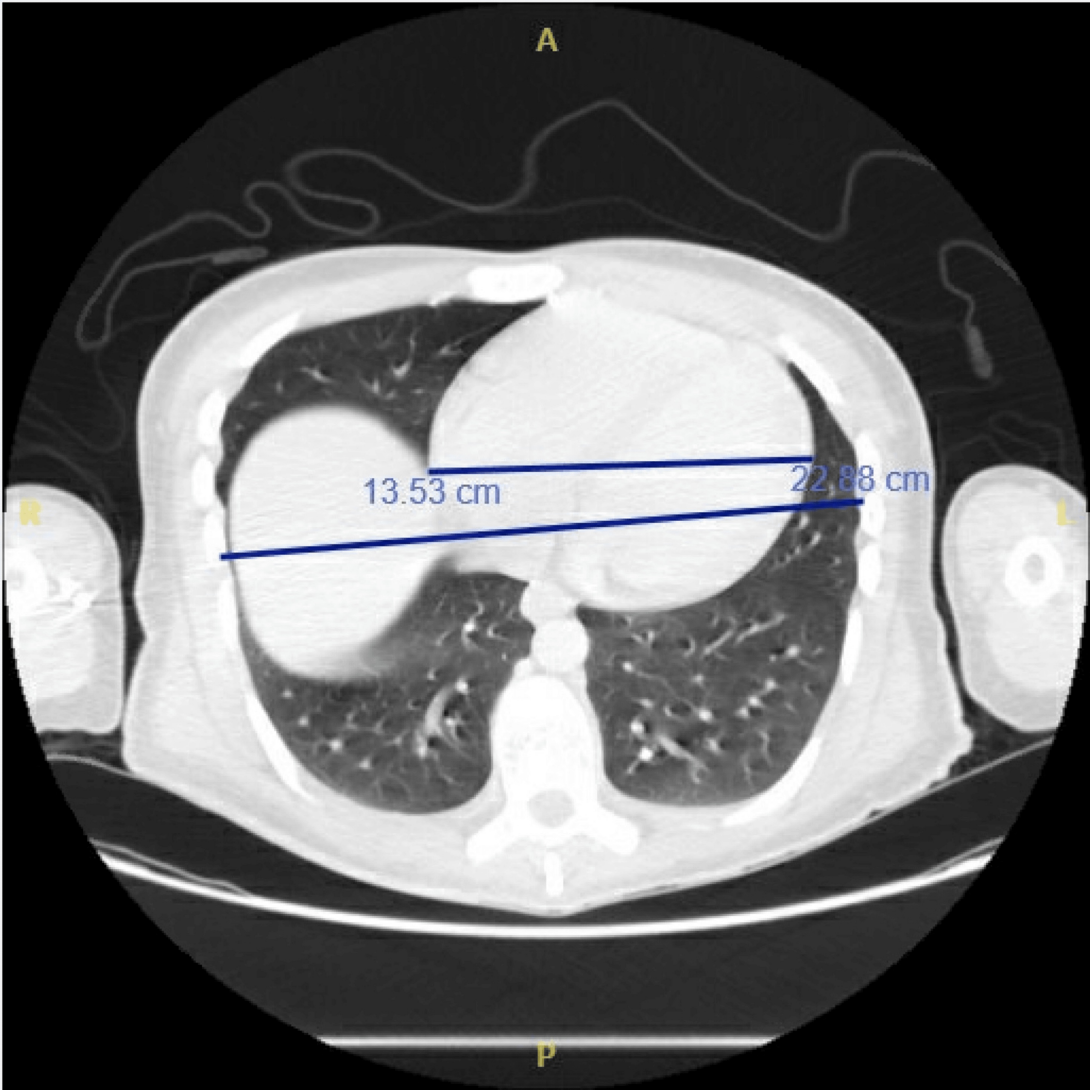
Axial contrast-enhanced chest CT showing mild cardiomegaly (cardiothoracic ratio ≈ 0.59) and borderline aortic root dilatation (3.7 cm).
